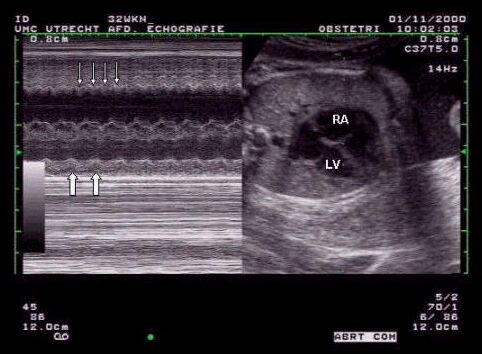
M-mode echocardiography of AF. Flutter contractions of the atrium are indicated by the small arrows; atrioventricular conduction is 2:1 and ventricular contractions are indicated by the large arrows.
